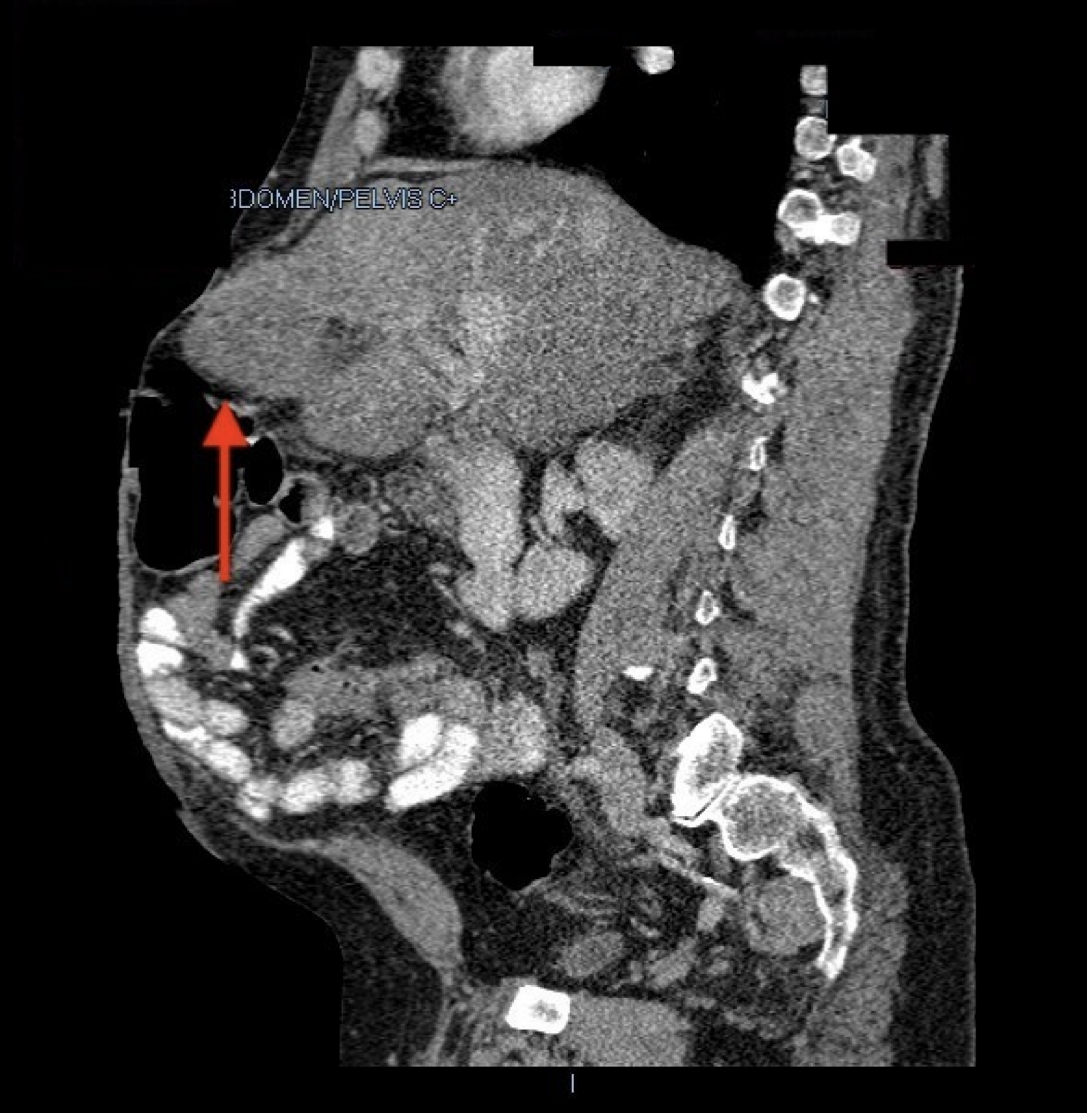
CT abdomen (sagittal view) showing herniation of the left hepatic lobe through an incisional abdominal hernia.
